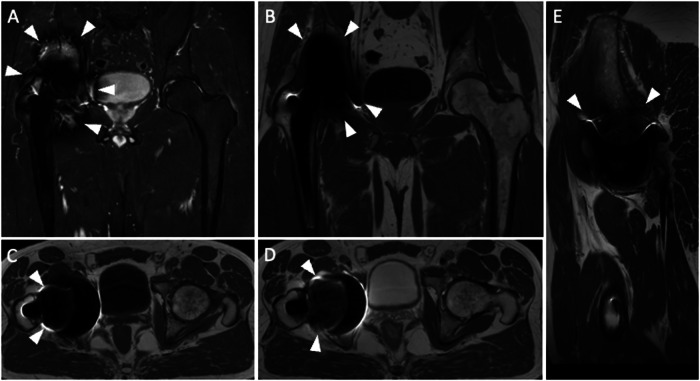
Pelvis MARS MRI of a 71-year-old male with right THA. Coronal STIR (A), coronal (B), axial (C) T1-weighted, axial (D), and sagittal (E) T2-weighted images are poorly diagnostic due to artifacts and incomplete fat suppression (both indicated by arrowheads) in the periprosthetic areas, particularly around the acetabulum. These artifacts could not be removed even using MARS sequences
